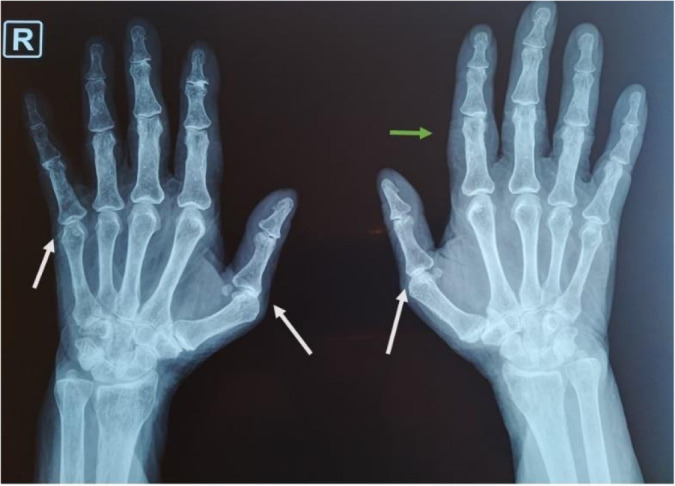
X-Ray shows ulnar deviation of both hands, subluxations of the 1st and 5th MCP joints (white arrows), soft tissue enlargement (green arrow) and absence of bone erosions.
